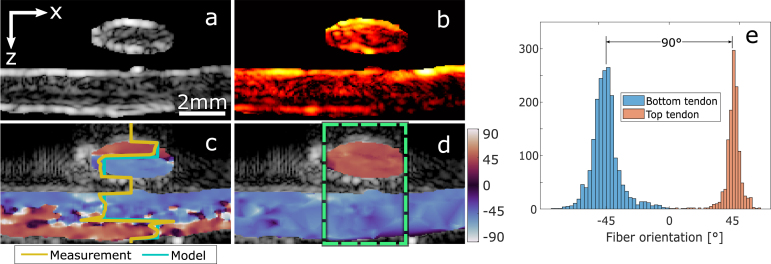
Analysis of stacked tendons perpendicularly oriented. (a) is the Ultrasound image, and (b) is the mean PA image. (c) is the phase detection for the two layers. The profile plot shows the behavior of the DS-PA phase along with a model-based profile of a perfectly homogeneous tissue for visualization. (d) is the corrected estimation of the fibers’ orientation. (e) shows the histograms for the two tendons within the region indicated by the dashed square. The peak of the distributions indicates the mean fiber orientation for the tendon.
